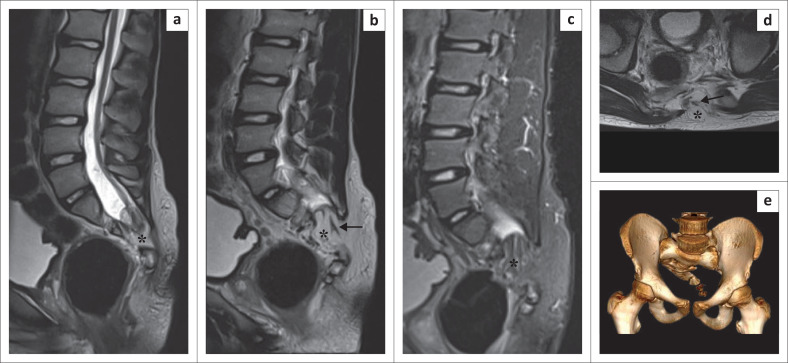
Lipomyelocoele. Sagittal T2-weighted image (T2WI) (a) shows a low-lying spinal cord tethered to a lipoma (*). The lipoma is extending into the spinal canal and is continuous with the subcutaneous fat posteriorly on sagittal T2WI (b), sagittal short tau inversion recovery (STIR) (c) and axial T2WI (d). Sagittal and axial T2WI (b, d) show the cord–lipoma interface (black solid arrow) at the level of the neural arches, without widening of the subarachnoid space. Volume rendered CT image (e) demonstrates a deformed sacrum.
